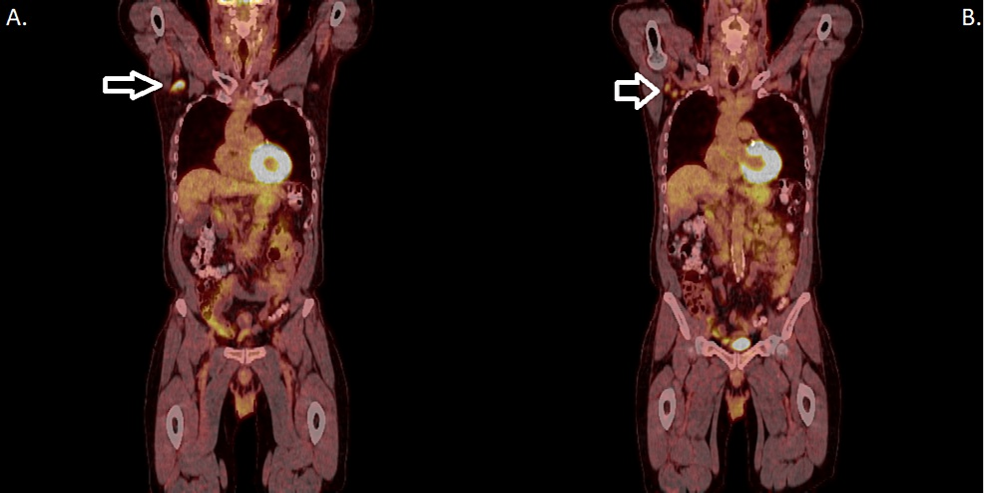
PET CT scan with FDG uptake A highlights the greatest area of uptake while B highlights the three nodes of interest. PET: positron emission tomography, FDG: fluorodeoxyglucose
